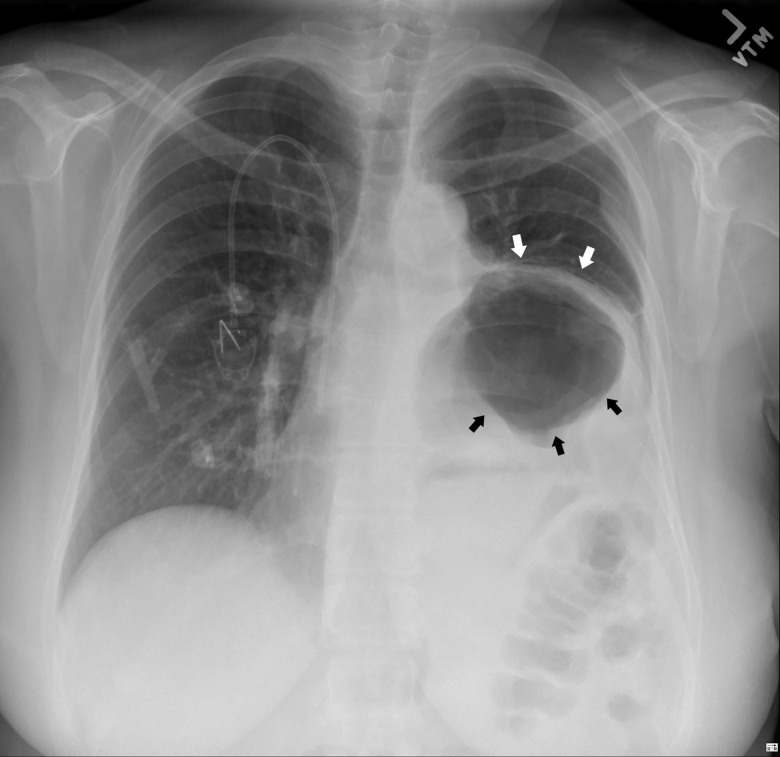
Chest radiograph showing an air-filled, thick-walled structure (black arrows) overlying the left lower thorax with marked elevation of the left hemi-diaphragm (white arrows).
